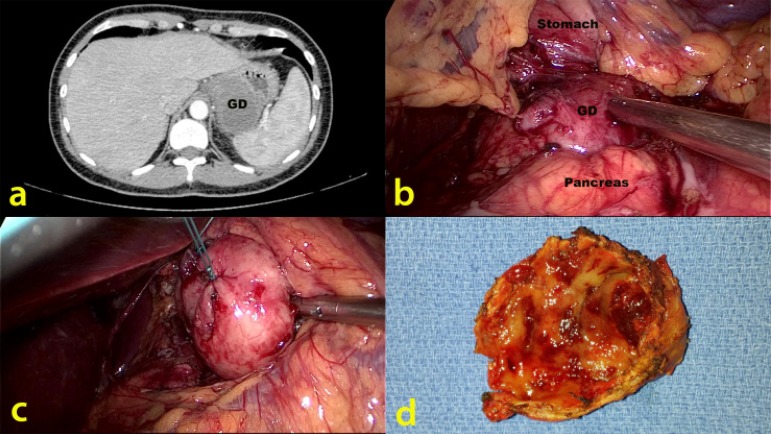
Single-port laparoscopic resection of gastric duplication: a) CT scan discloses a
